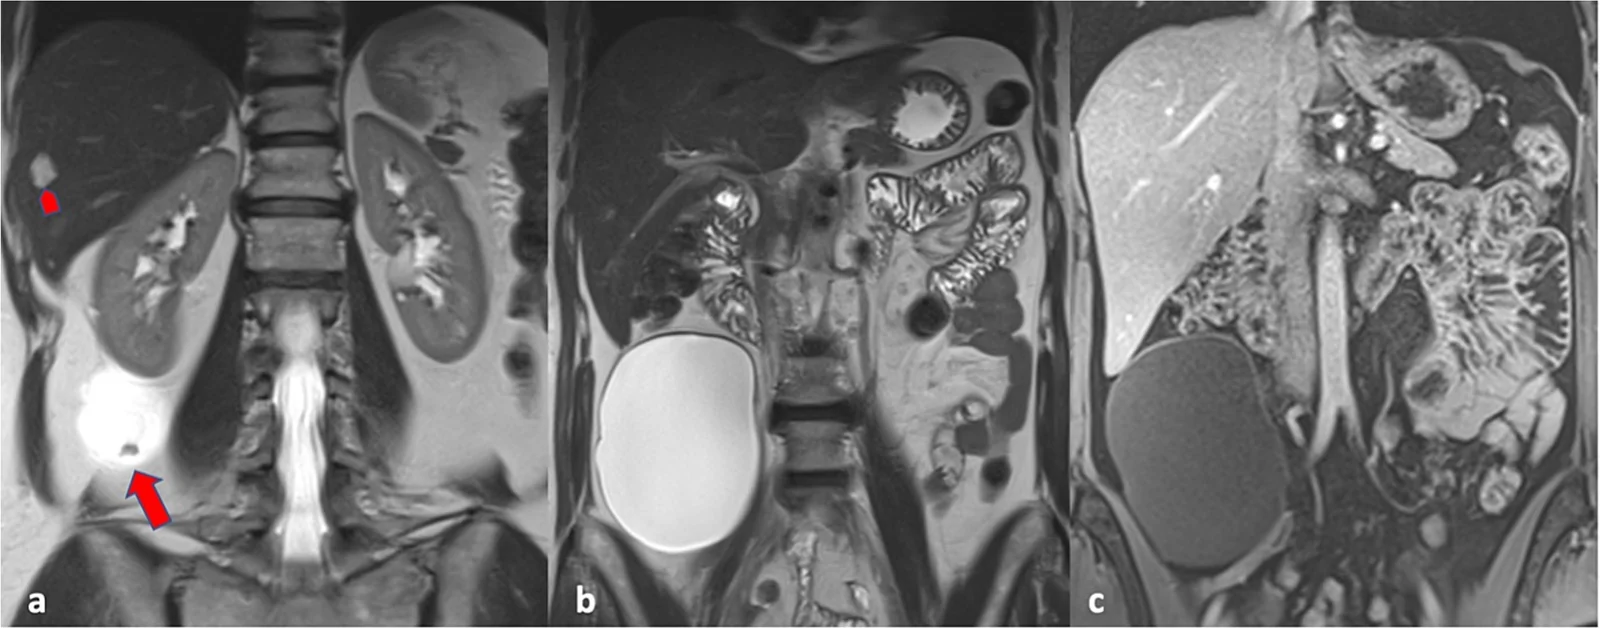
(a–c) MRI: coronal T2 WI (a, b) and T1 WI after i.v. contrast administration. (c) The large cystic mass is hyperintense and hypointense on T2 WI and T1 WI, respectively. Its wall is thin and well-defined without evidence of internal septations or papillary projections. A small peripheral hypointense calcified nodule is noted (big arrow). There is only mild peripheral enhancement after i.v. contrast administration. A small hemangioma is demonstrated at the right liver lobe (small arrow).
